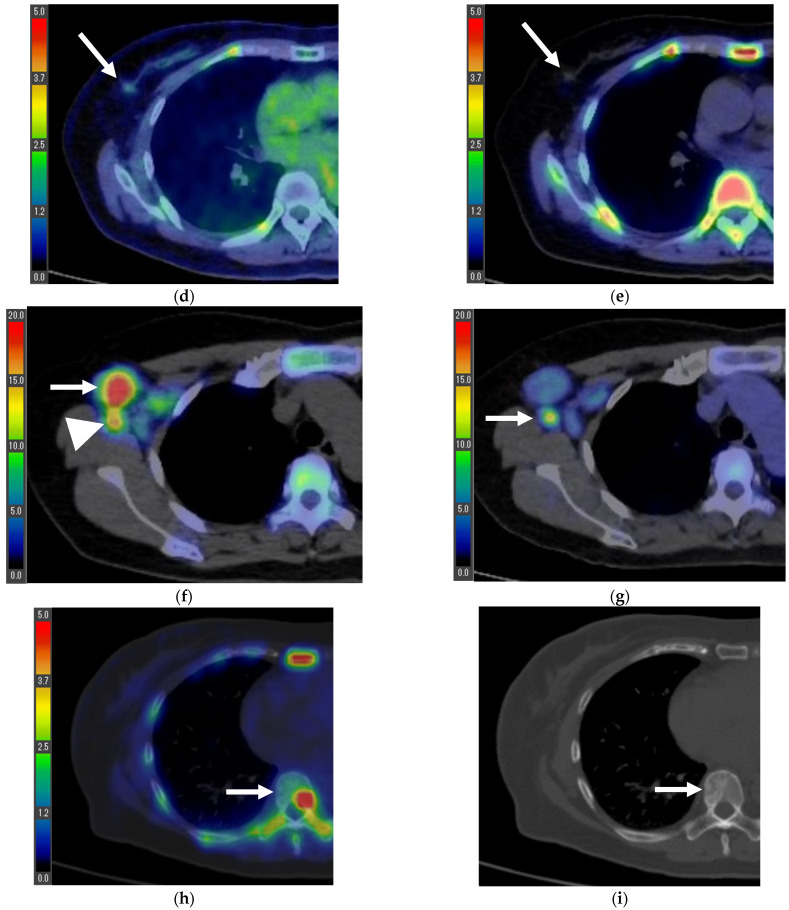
First maximum intensity projection (MIP) of ^18^F-FDG PET (a), MIP of ^18^F-FLT PET performed 3 days later (b), and MIP of ^18^F-FLT PET performed 5 months later (c). In the axial image of the PET/CT at the slice of the primary right breast cancer, both the ^18^F-FDG PET/CT and ^18^F-FLT PET/CT showed trace accumulation ((d,e), arrows). The axillary lymph node with the greatest accumulation differed between the ^18^F-FLT PET/CT (f) and ^18^F-FDG PET/CT (g), and the SUVmax values were 30.0 and 14.2, respectively (arrows). The lymph node corresponding to the node with the greatest accumulation on the ^18^F-FDG PET/CT showed an SUVmax of 16.7 on the ^18^F-FLT PET/CT ((f), arrowhead). The post-chemotherapy thoracic spine metastasis showed low ^18^F-FLT accumulation and bone sclerosis, suggesting that cell proliferation had decreased ((h,i), arrows). Patient 4 (Figure 2).
